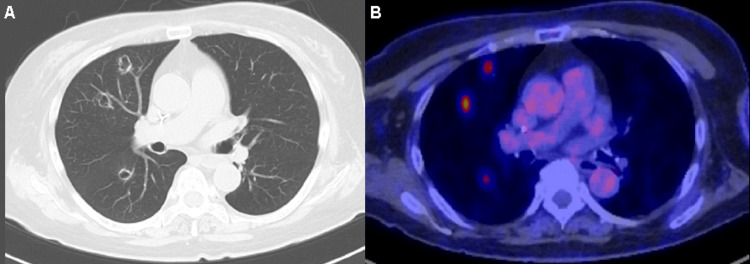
Radiological findings of the tumor in Case 2 before nivolumab administration. Findings before nivolumab administration. Computed tomography (CT; A) and fluorodeoxyglucose positron-emission tomography (FDG-PET; B) revealed multiple cavernous nodules in the right lung field.
